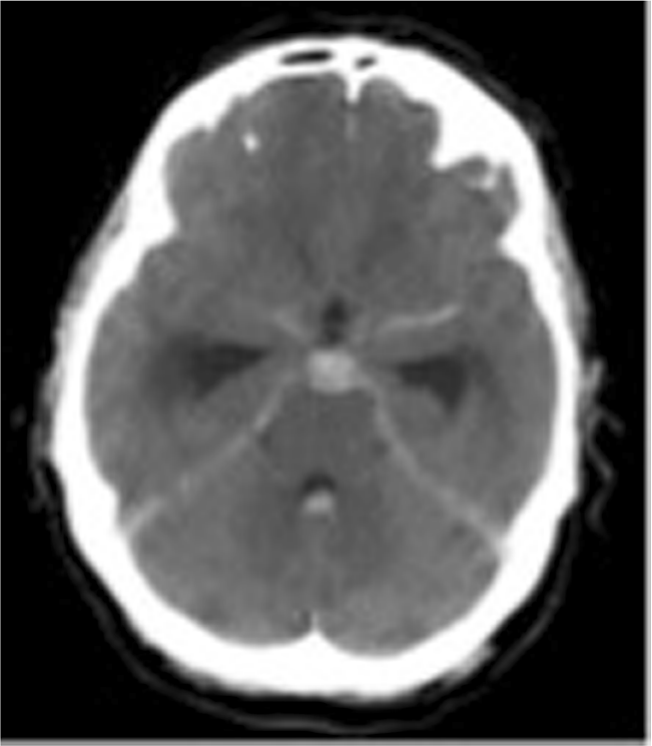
CT brain scan demonstrating subarachnoid blood concentrated in the basal cisterns and fourth ventricle and ventriculomegaly
